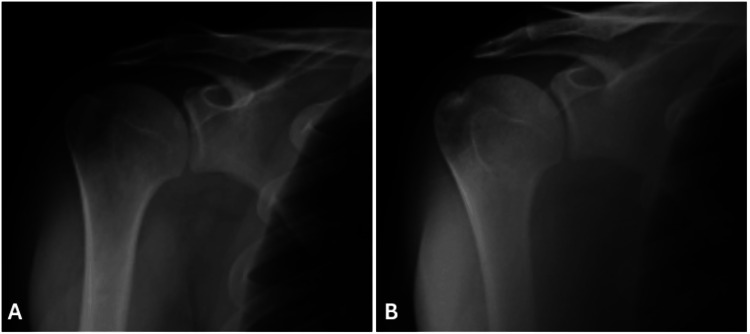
The true anteroposterior radiographs taken immediately post-trauma (A) and at the three-month follow-up (B) demonstrate a normal subacromial space (measured at approximately 10mm).
